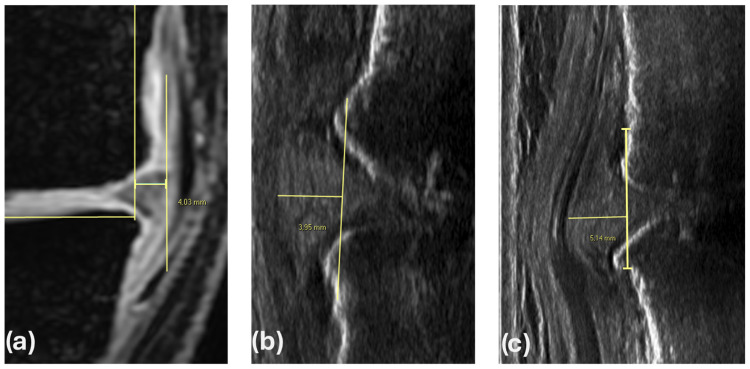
Measurement of meniscal extrusion in a male patient (51 years) using magnetic resonance (a), ultrasounds in the clinostatic position (b) and ultrasounds in the orthostatic position (c).
